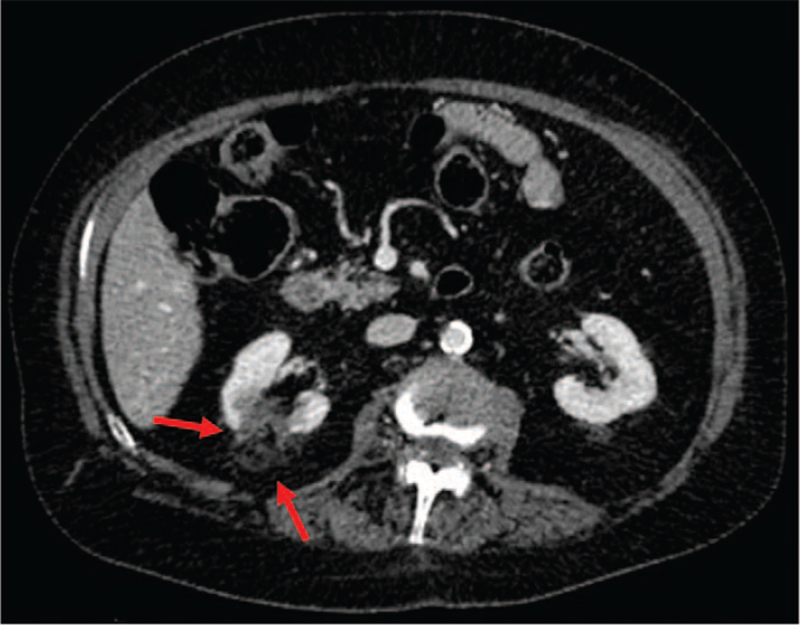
Post-treatments CT demonstrating complete response according to mRECIST. Please, note the disappearance of intratumoral vascular enhancement in the target lesion.
